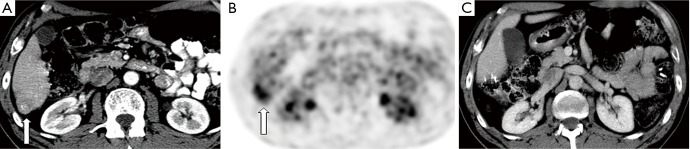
A 64-year-old female s/p right hemicolectomy for adenocarcinoma presenting with solitary segment 6 metastases discovered 3 years post-operatively on routine surveillance imaging. (A,B) Axial contrast enhanced CT image and corresponding PET image demonstrating FDG-avid segment 6 liver metastasis (arrow); (C) post-operative axial CT image obtained 8 years after laparoscopic liver resection demonstrating no residual or recurrent disease.
